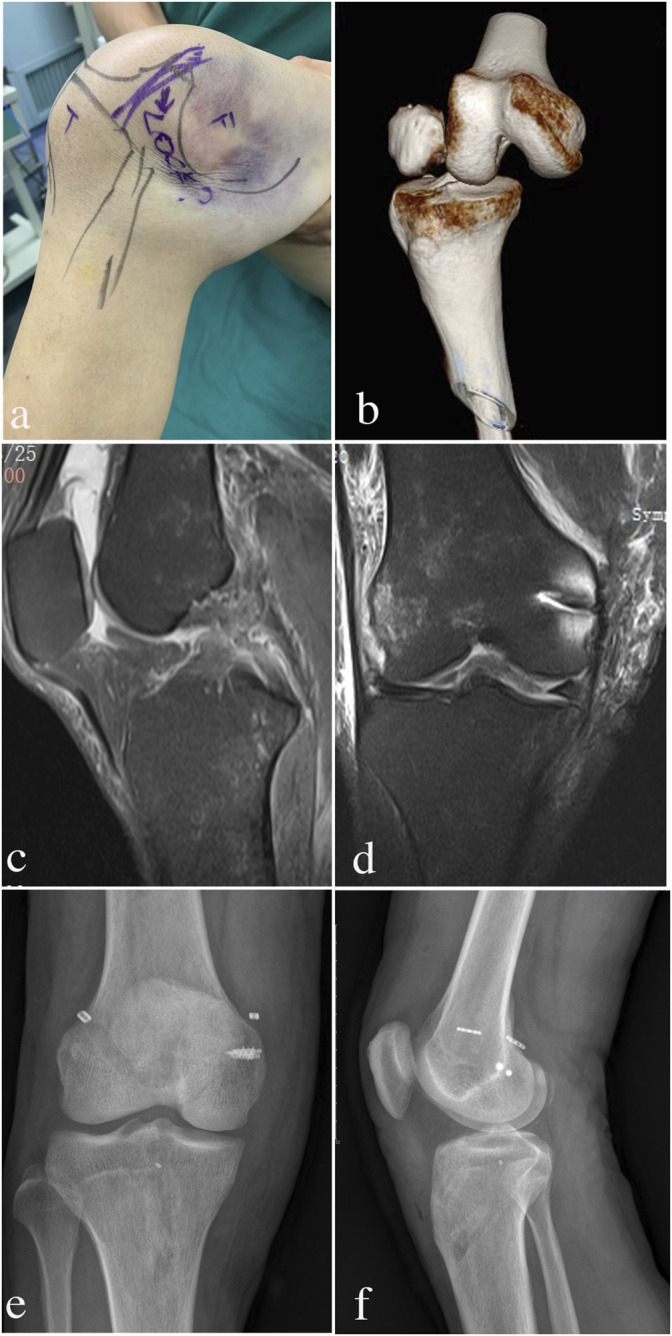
Evolution process of IKD patient appearance and imaging examinations from injury to postoperative follow-up. (A) “Dimple sign” and severe ecchymosis with invagination of skin and soft tissue can be observed on the medical side; (B) before the stage-1 operation, CT showed the knee posterolateral dislocation; (C): before stage-2 operation, MRI showed that the MCL structure had been repaired; d: before stage-2 operation, MRI showed the ACL and PCL rupture; (E,F): X-ray showed normal knee alignments at 0.5 years and 1 year follow-up.
